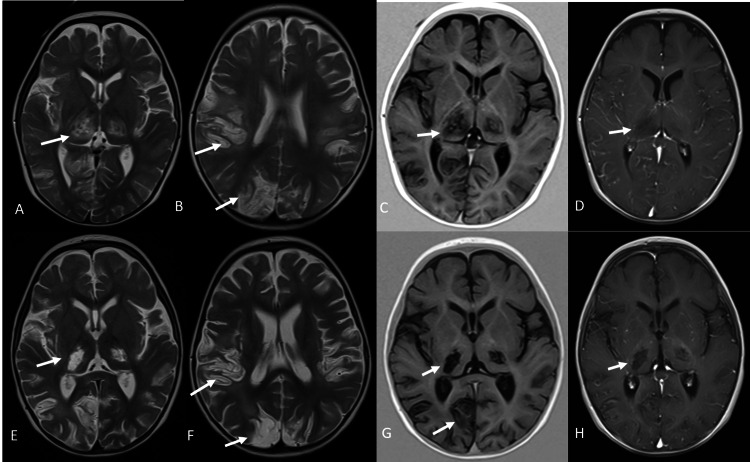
First and second brain MRI Axial T2-weighted images (A and B) and the axial T1-inversion recovery image (C) show necrosis of both the thalamus and at the cortico-subcortical junction of both parietal and occipital lobes with a lack of contrast enhancement (D). Images E-H show the evolution of the previous findings two weeks later with the loss of parenchyma MRI: magnetic resonance imaging
